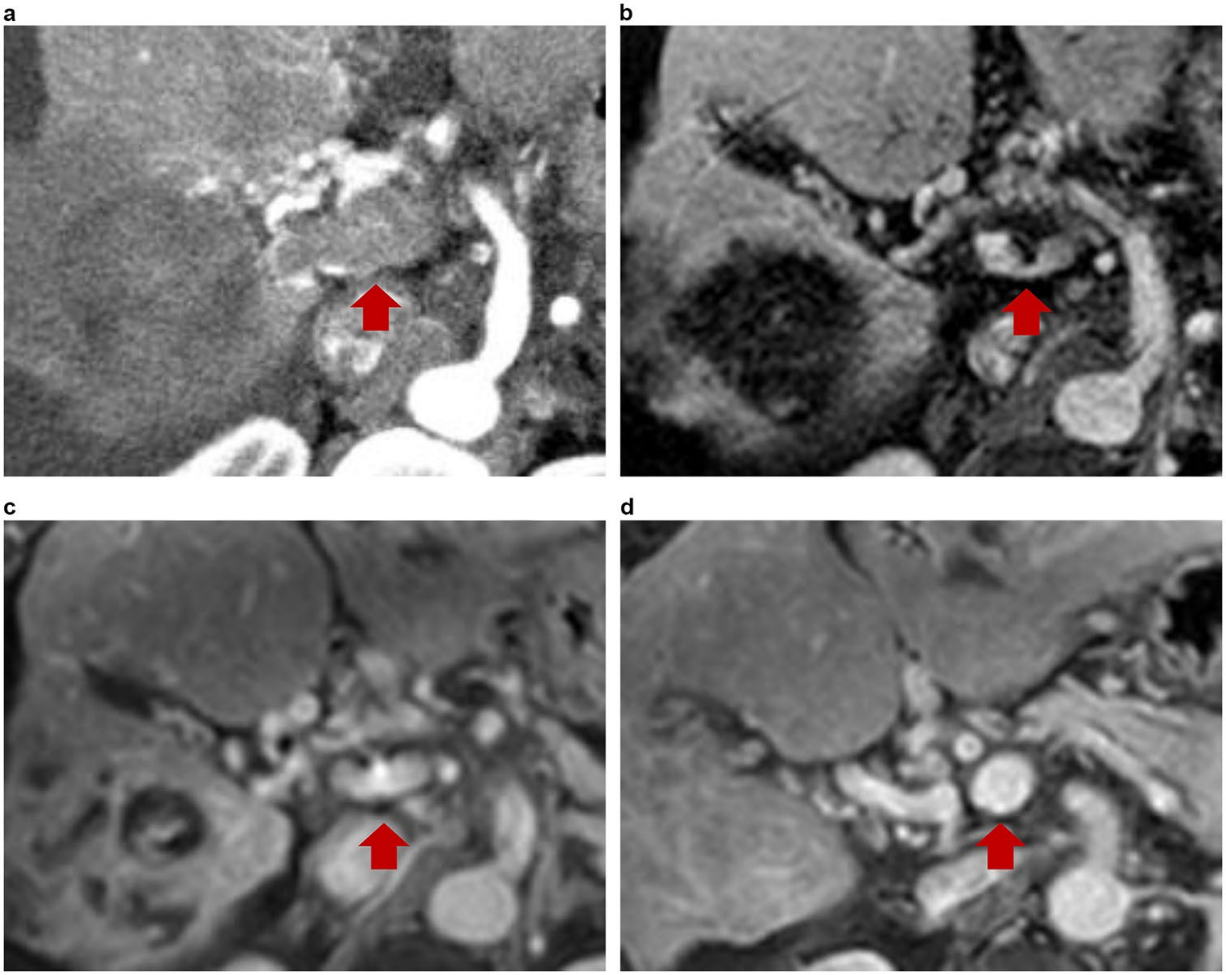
Serial magnetic resonance imaging of a 65-year-old male with hepatocellular carcinoma (HCC) with main portal vein tumor thrombus (PVTT) treated with a PD-1 inhibitor (a) before treatment, the thrombus was nodular, expanded, and enhanced in the main portal vein (arrow in a). Scans taken 2 (b), 5 (c), and 13 (d) months after treatment, showing marked PVTT regression
